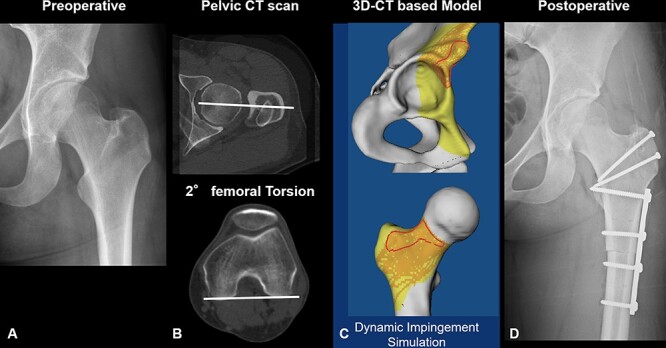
Preoperative AP radiograph (A), measurement of FV (B) and 3D-CT-based model of the pelvis and the proximal femur (C) showing anterior intra- and extraarticular hip impingement conflict of a 20-year-old male patient who underwent subtrochanteric rotational femoral osteotomy to increase FV combined with SHD (D).
